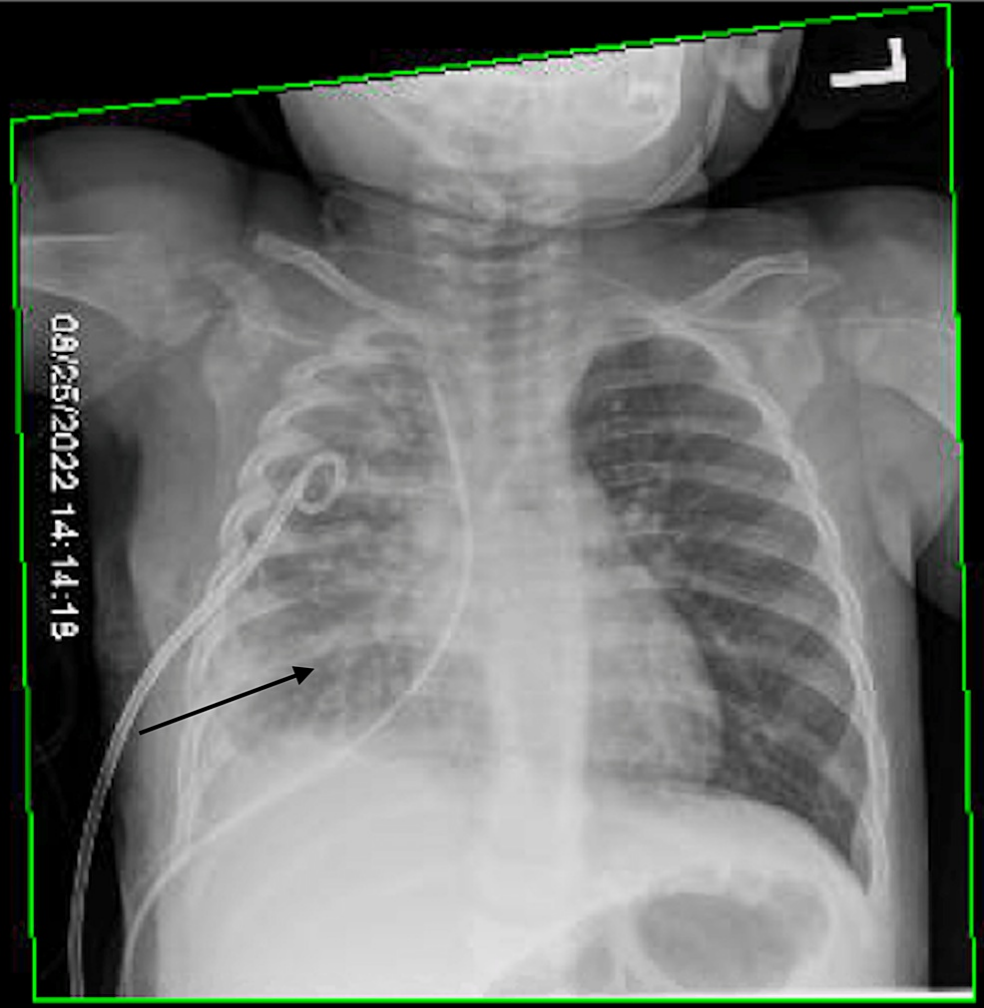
Chest X-ray showing right-sided pleural effusion (black arrow).
